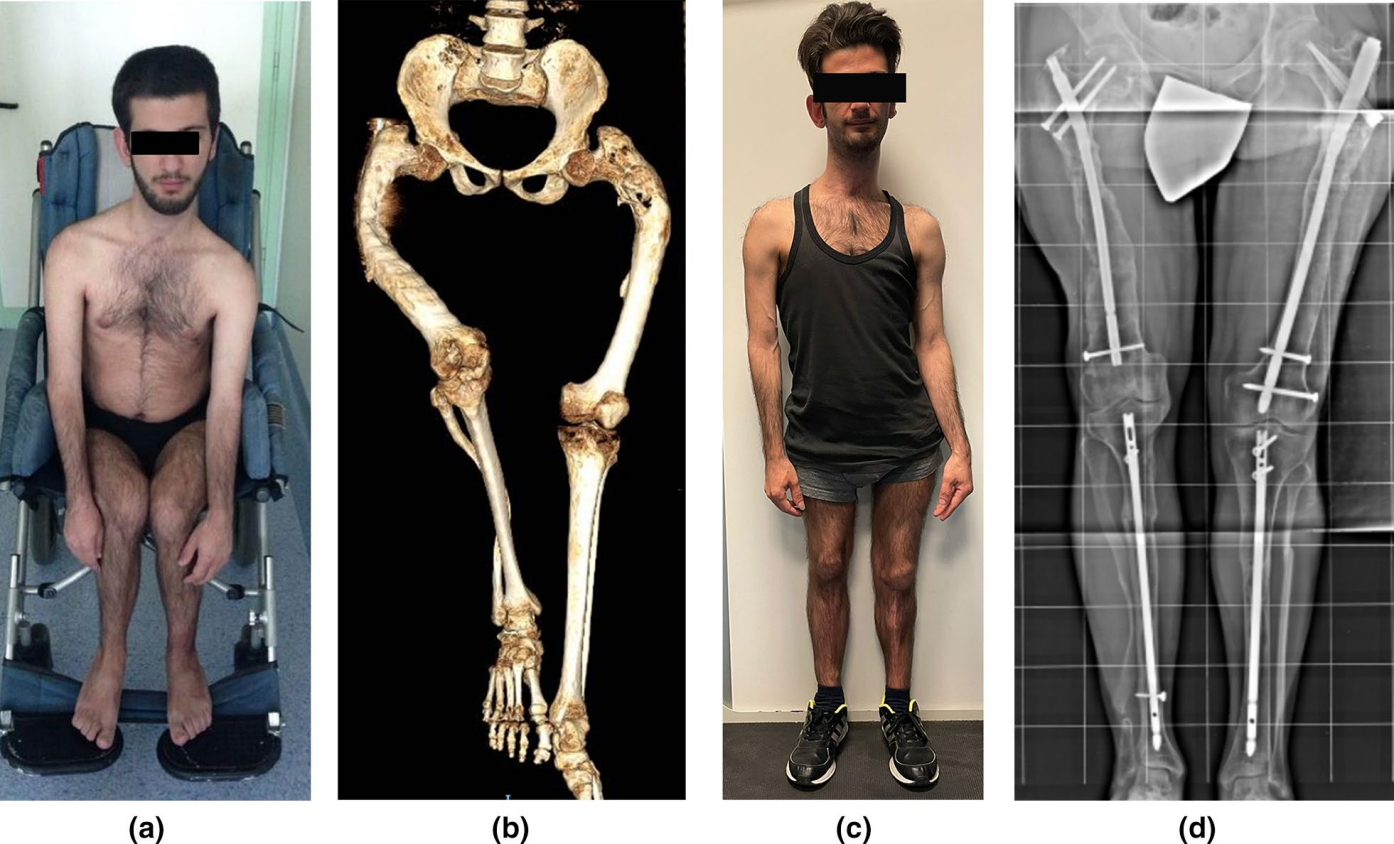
a Eighteen-year-old boy with McCune–Albright syndrome and bimelic fibrous dysplasia involvement. He was wheelchair dependent, with a clinical Jung score of 0 points. He had had a non-operative orthopedic treatment for several lower limb fractures. b Three-dimensional CT scan of the pelvis and lower limbs showing a severe bilateral shepherd’s crook deformity (type 6 femoral deformity) with 6 cm of lower limb length discrepancy. c At follow-up, 5 years after the last intramedullary nailing, he walked independently with a mild painless limp; he was able to perform all the activities of daily living and he had a normal social life, regardless of the 2 cm of both lower limb length discrepancy and knee height asymmetry (d). An excellent result was obtained, with a clinical Jung score of 9 points
